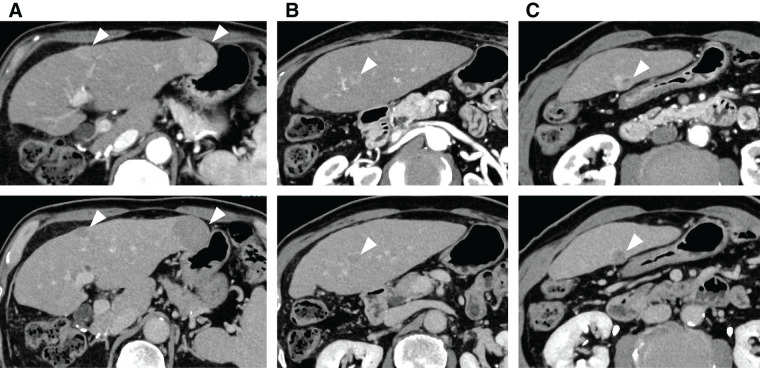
Abdominal contrast-enhanced CT images taken before atezolizumab plus bevacizumab therapy (above: arterial phase images; below: equilibrium phase images). (A) A 14-mm HCC in S3 and a 35-mm HCC lateral to S2 are detected. (B) A 10-mm HCC in the middle part of S2 is detected. (C) A 13-mm HCC in the caudal part of S2 is detected. Four masses are identified in the lateral segment of the liver, showing hyperenhancement in the arterial phase (above images in A–C) and washout in the equilibrium phase (below images in A–C), and are diagnosed as HCCs. HCC, hepatocellular carcinoma
